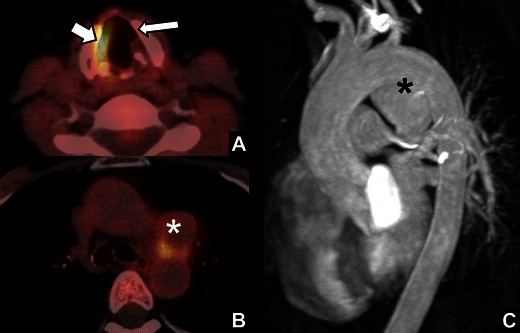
Aortic arch aneurysm.* A 44-year-old patient with a history of systemic lupus erythematosus and herpes zoster infection presenting with symptoms of left VCP. Because of the patient’s history, a FDG-PET with low-dose CT was obtained. a Fused PET-CT image at the level of the cricoid showed widening of the left laryngeal ventricle (*long arrow*), note the increased FDG-uptake in the unaffected right vocal cord due to hypertrophy (*short arrow*). b Images at the level of the aortic arch showed a mass in the AP-window without FDG uptake (*). c Contrast-enhanced MR angiography of the aortic arch showed a saccular aneurysm (*) causing compression of the vagal nerve at the site of the branching of the left laryngeal recurrent nerve, thereby causing VCP
